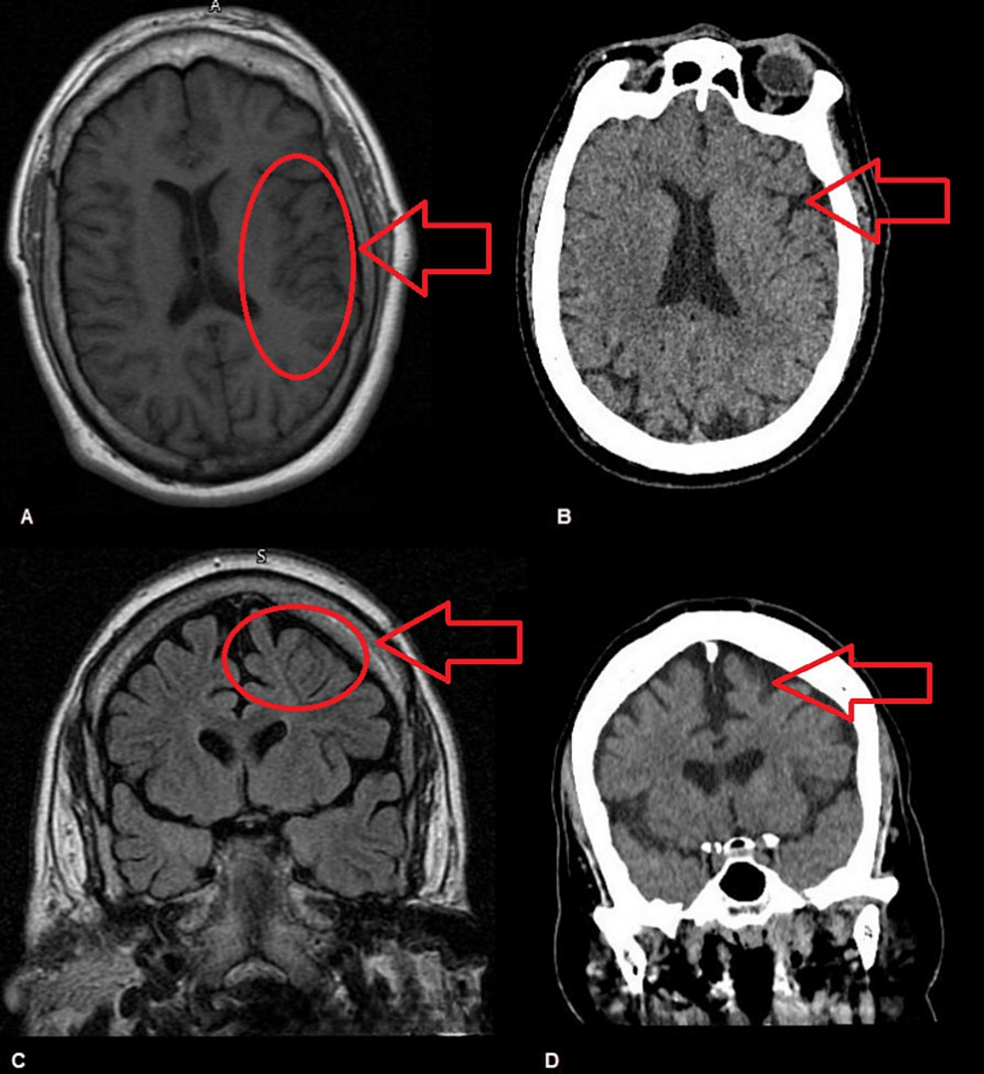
Cranial non-contrast computed tomography scan. (A, B) Axial view showing left temporal lobe atrophy. (C) Coronal view from 2007 showing left frontal lobe sulci. (D) Coronal view from 2023 showing left frontal lobe with wide sulci, fewer in number.
